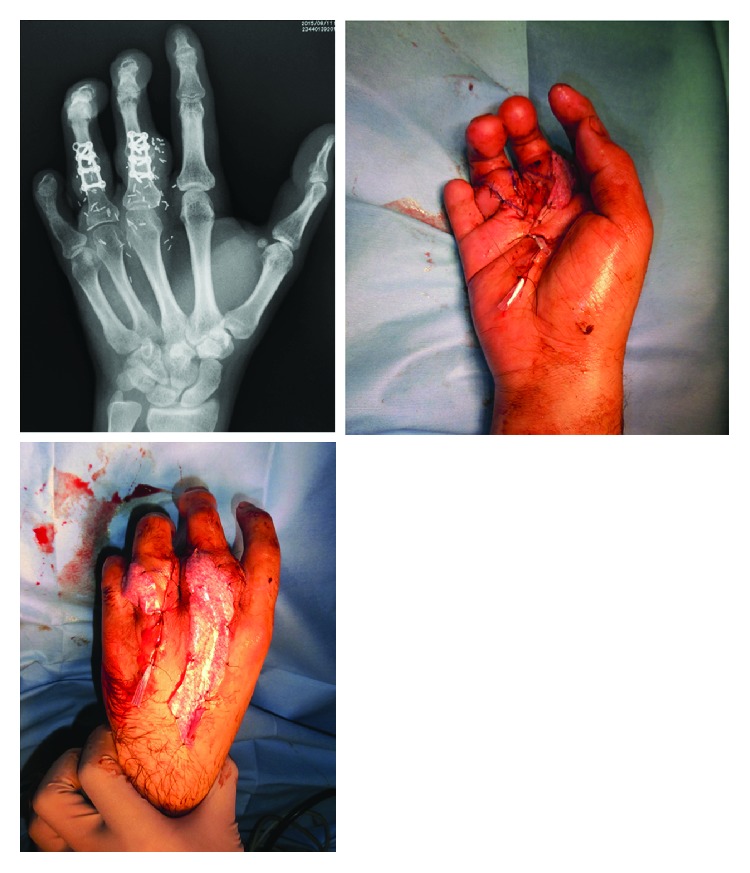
X-ray of the hand after transplantation. The second toe and proximal phalanx are fixed with a plate.
